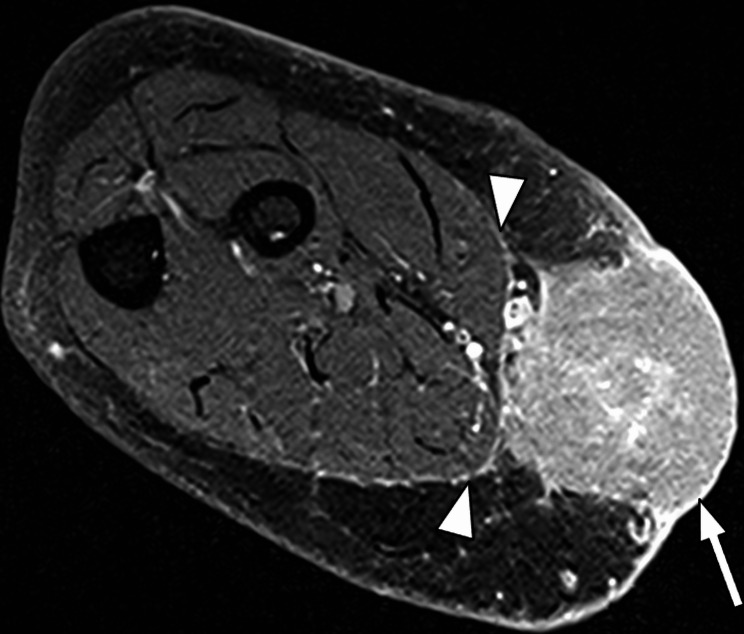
Transversal contrast enhanced fat-saturated T1-weighted image of a 55-year-old female shows a subcutaneous tumor (arrow) of hyper intense signal intensity and associated enhancement of adjacent fascia (arrowheads)
